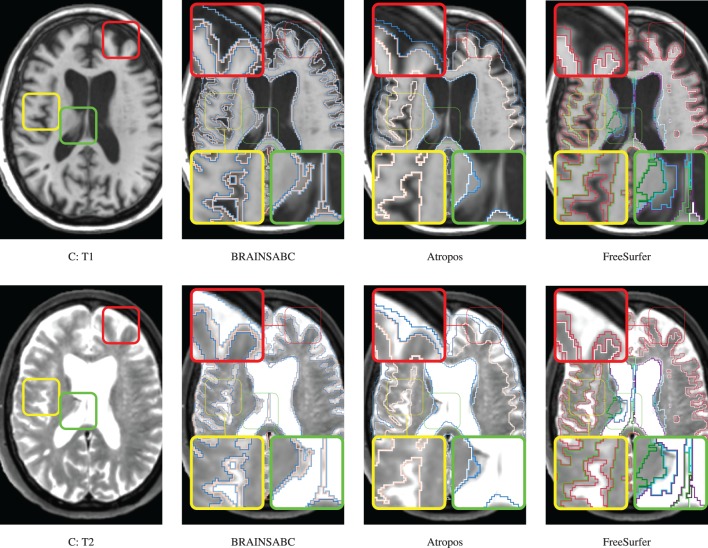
Visual Comparison of Scan C in Table 2. Tissue classification results from three applications, BRAINSABC, Atropos of ANTS Tools, and FreeSurfer on top of T1- and T2-weighted images, multi-modal processing. This subject present relatively small tissue ratio, reflecting brain atrophy progression to some extent. BRAINSABC and other two methods produced very similar results in this scan. Differences between methodologies are also observed as highlighted in red, yellow, and green boxes. Red box contrasts classification on the cortical region, where BRAINSCut draws a nice borderline for surface CSF in regarding to both T1- and T2-weighted images. Green box magnified CSF and GM border on the subject with enlarged ventricles where T1-weighted image shows artifacts. Last, yellow box also shows different classification results between tools. Again, CSF classification present some disagreement between FreeSurfer and BRAINSABC (green box).
